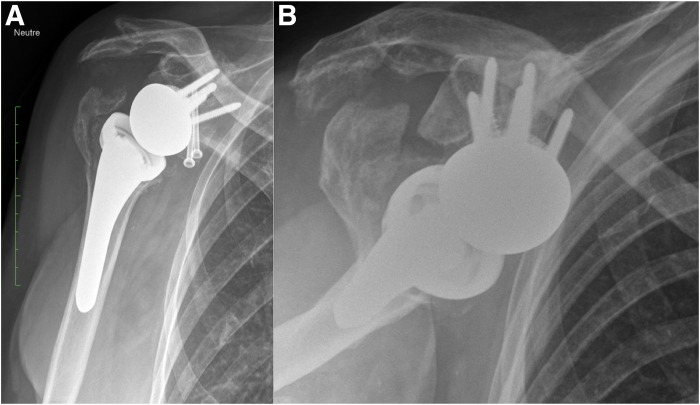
Anteroposterior (A) and lateral (B) radiographic views of the right shoulder at 9 months postoperatively, demonstrating maintained position and integration of the baseplate, coracoid graft, and posterior tricortical iliac crest autograft.
